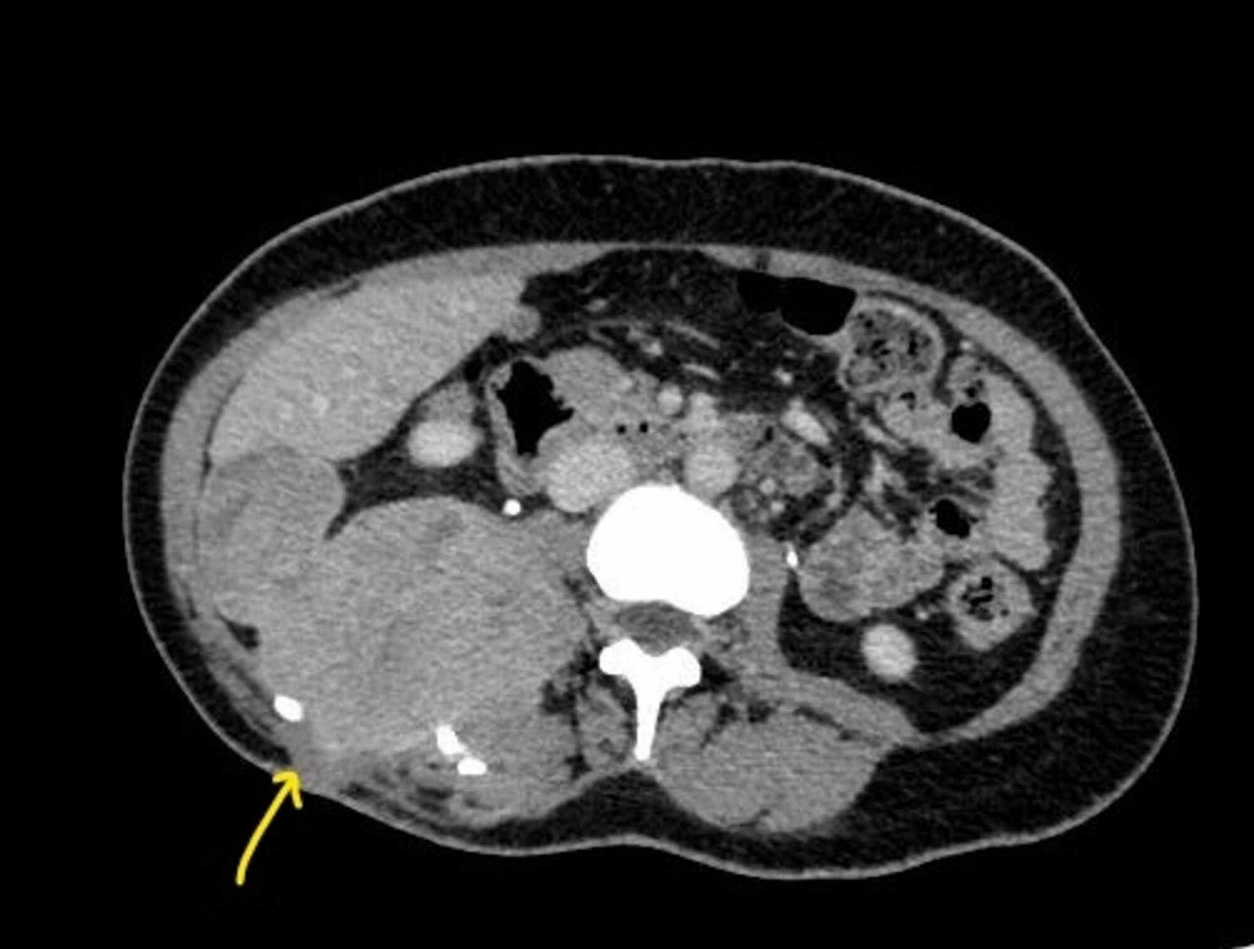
Contrast-enhanced CT image of tumor extending to posterior abdominal wall.
